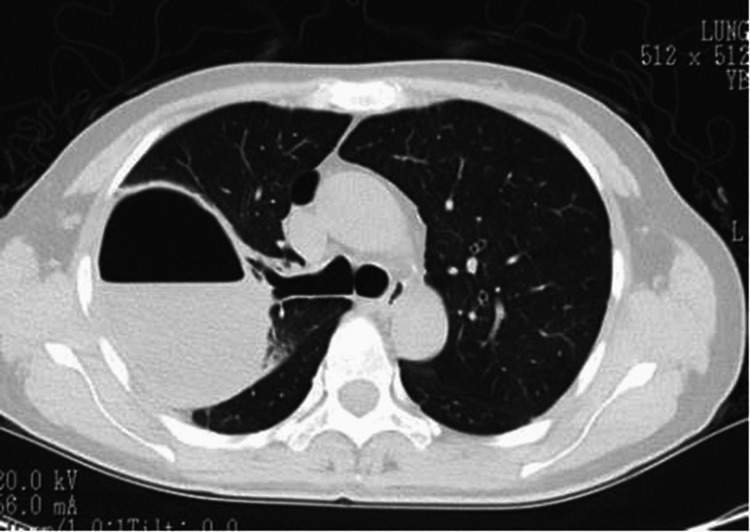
Axial CT showing progression to abscess A thick-walled cavity with pericystic infiltration and fluid level in the right upper lobe is seen, consistent with an abscess.
